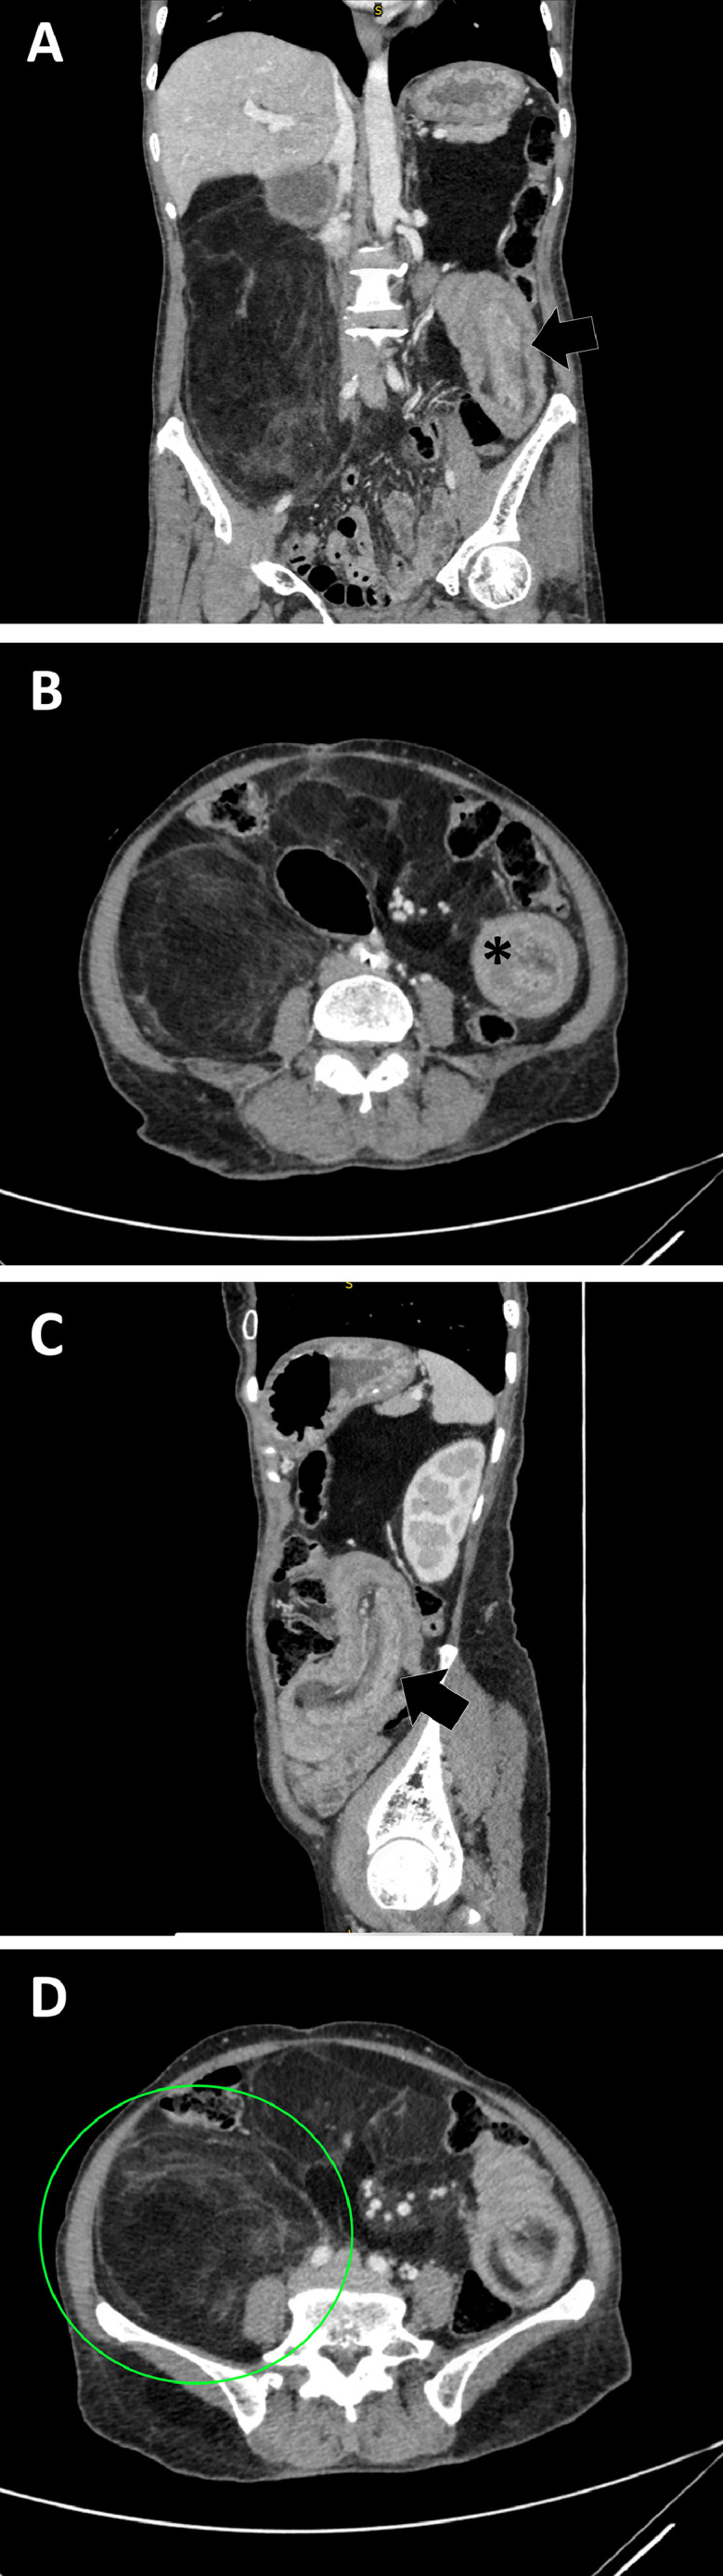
CT abdomen with coronal (A) and axial (B) view showing small bowel intussusception with sausage-like appearance (black arrow) and target sign (asterisk) where fat and mesenteric of small bowel seen within the lumen. It also shows a large right retroperitoneal mass encasing part of the posterior surface of the right kidney. CT abdomen with sagittal (C) view showing a more obvious small bowel intussusception with fat and mesenteric content (black arrow). CT abdomen with axial (D) view showing a different level of cut at L2 level showing the right retroperitoneal mass (green circle) encasing the whole right kidney. It also shows multiple intraperitoneal liposarcoma with similar CT features.
